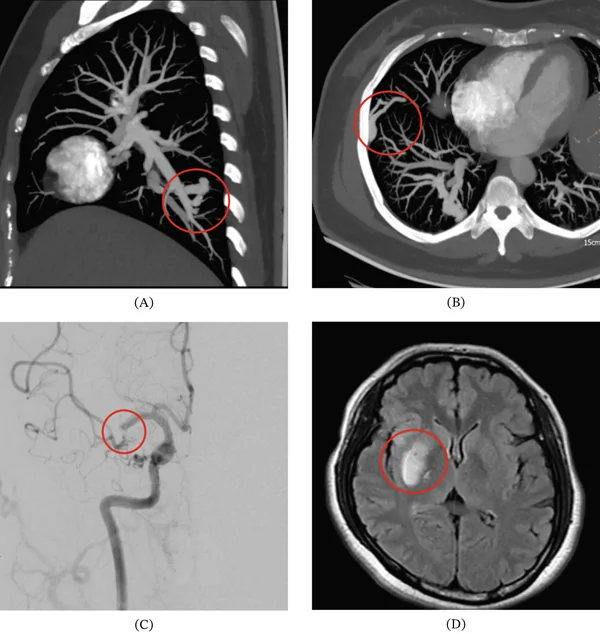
Images of the proband′s aunt in Family 4. (A) Sagittal plane MIP shows communication between the posterior basal segment of the right lower lobe and the right inferior pulmonary vein. (B) Axial plane MIP shows a vascular‐like enhancing nodule in the lateral segment of the right middle lobe. (C) Right intracranial artery DSA shows partial occlusion of the M2 segment of the right middle cerebral artery with formation of abnormal collateral vessels. (D) Head MRI, T2‐FLAIR shows abnormal signal in the right basal ganglia region.
